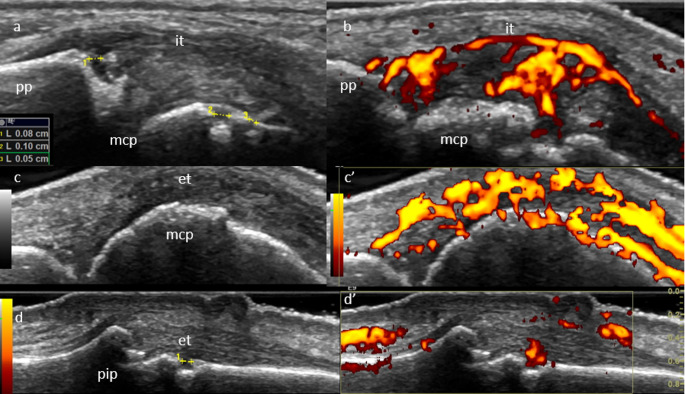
Ultrasound detection of early erosive changes in psoriatic arthritis. Legend.(a) Image showing multiple millimetric bone erosions (callipers) at the lateral aspect of the second metacarpophalangeal joint (longitudinal lateral view). (b) A “hot” bone erosion with a cortical break filled with synovial pannus (longitudinal lateral view). (c) Grey scale and (c’) power Doppler images showing close contact between synovial pannus and cortical bone with evident irregularities of the cortical bone (longitudinal dorsal view). (d) Grey scale and (d’) power Doppler images of the third proximal interphalangeal joint showing a “hot” bone erosion and active synovitis (longitudinal dorsal view). Acronyms: et: extensor tendon; it: interosseous tendon; mcp: metacarpophalangeal; pip: proximal interphalangeal
